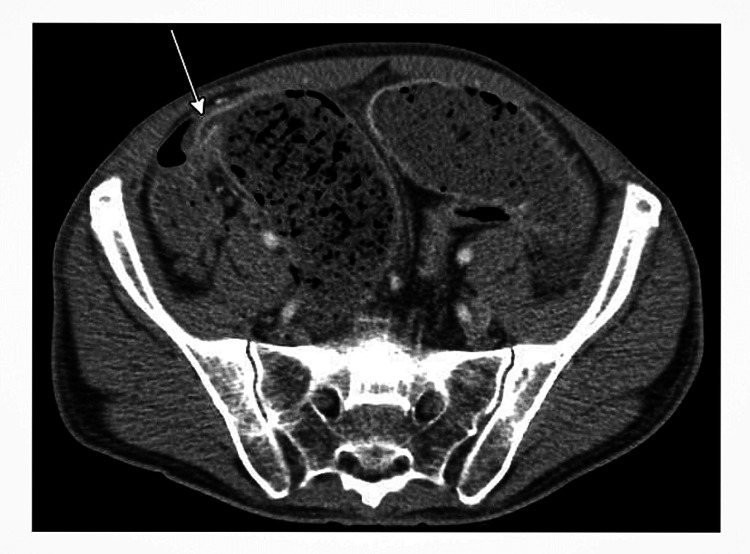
CT abdomen/pelvis with small bowel obstruction (white arrow pointing to the area of obstruction from terminal ileal stricture near the ileocecal junction).
